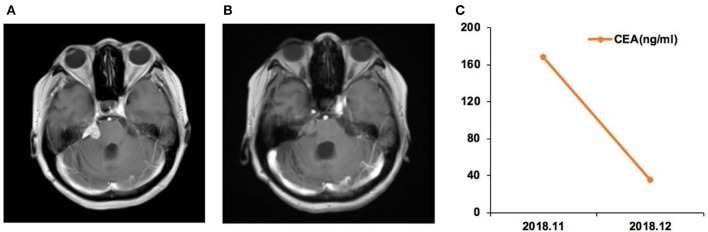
Case 12 was a female of 57 years of age, with lung adenocarcinoma but without smoking history. (A) In September 2018, the right cerebellopontine angle area, the edge of the tetras, and the lateral edge of the right arm were abnormally enhanced on magnetic resonance imaging. (B) In December, the enhancement intensity was decreased on the right side, and her condition was improved. (C) Carcinoembryonic antigen (CEA) levels before and after afatinib treatment.
